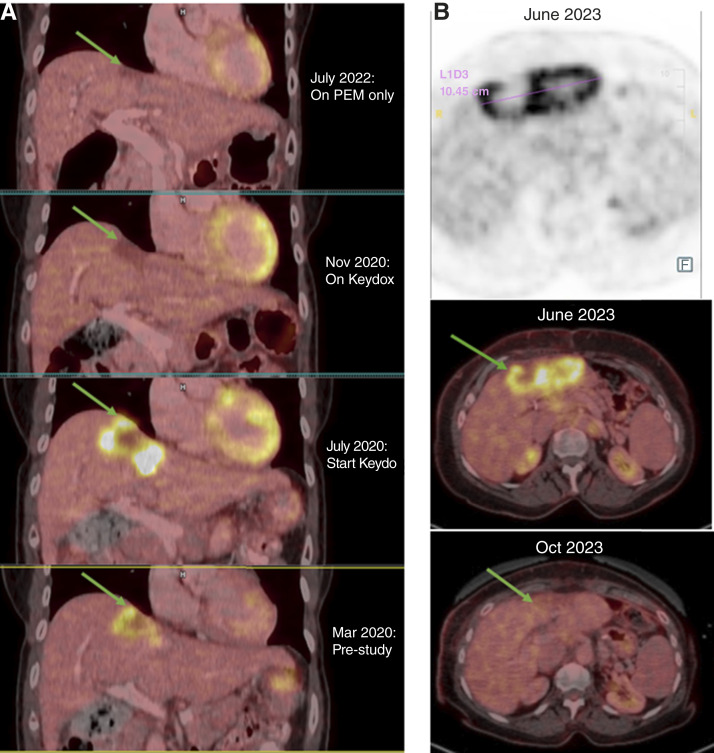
Response of hepatic metastasis to PLD + PEM in patients #10 (A) and #30 (B). A, This 75-year-old patient (see #10 in Supplementary Table S3; Supplementary Fig. S3) with a large liver metastasis had a complete and durable response. Bottom to top, PET-CT fusion images of a metastasis with significant progression between March and July 2020 (green arrows), followed by a major response in November 2020 under the Keydox regimen and a residual scar on July 2022 under single-agent PEM for the last 9 months. B, This 65-year-old patient (see #30 in Supplementary Table S3; Supplementary Fig. S9) with a large liver metastasis had a complete and durable response. Top to bottom, PET captures and fusion images of a 10.45-cm metastasis (pink line) at start of Keydox treatment (green arrow) with a complete regression 4 months later, maintained with PEM-only treatment for 12 months.
